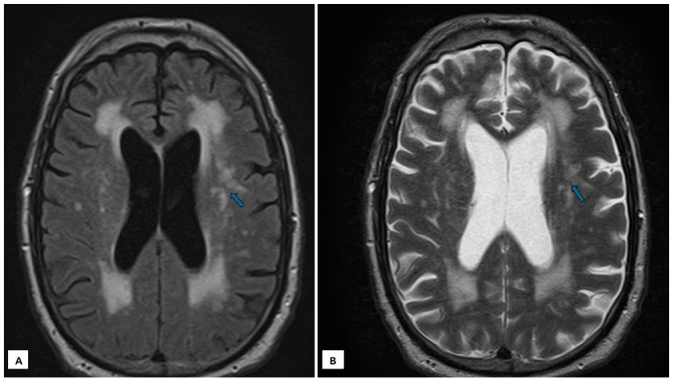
(A) Flair magnetic resonance imaging (MRI) of the brain showing cerebral small vessel disease. (B) T2 MRI of the brain showing cerebral small vessel disease.
